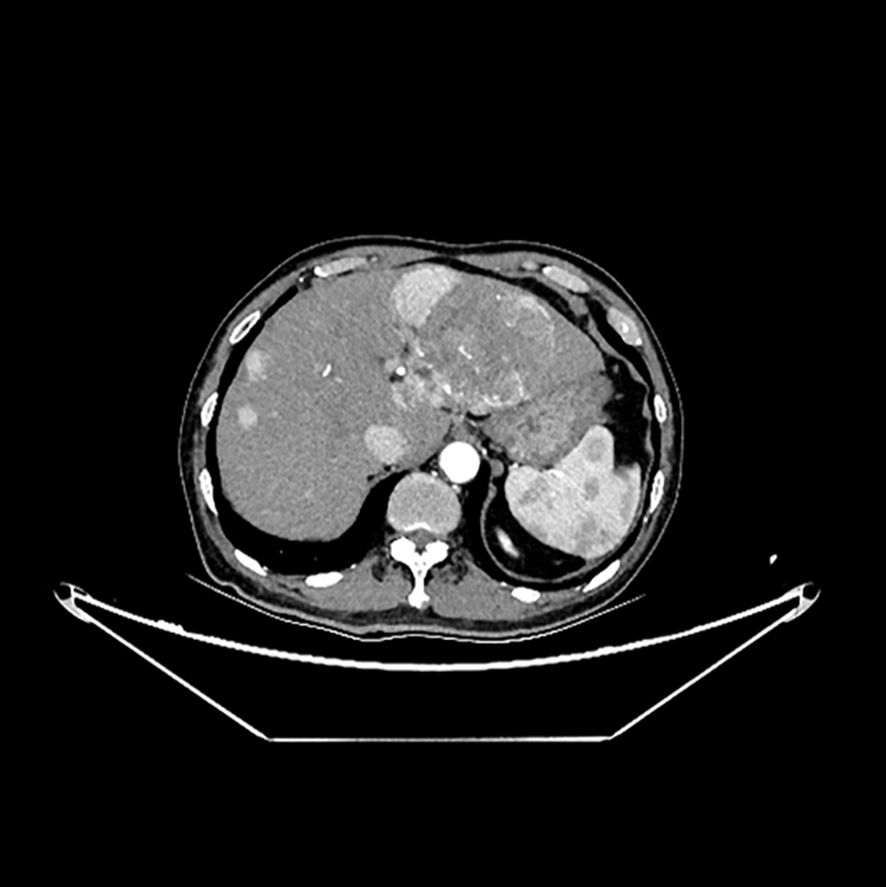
Tumor lesions in his left liver and normal outline of abdominal aorta in the computed tomography examination.
